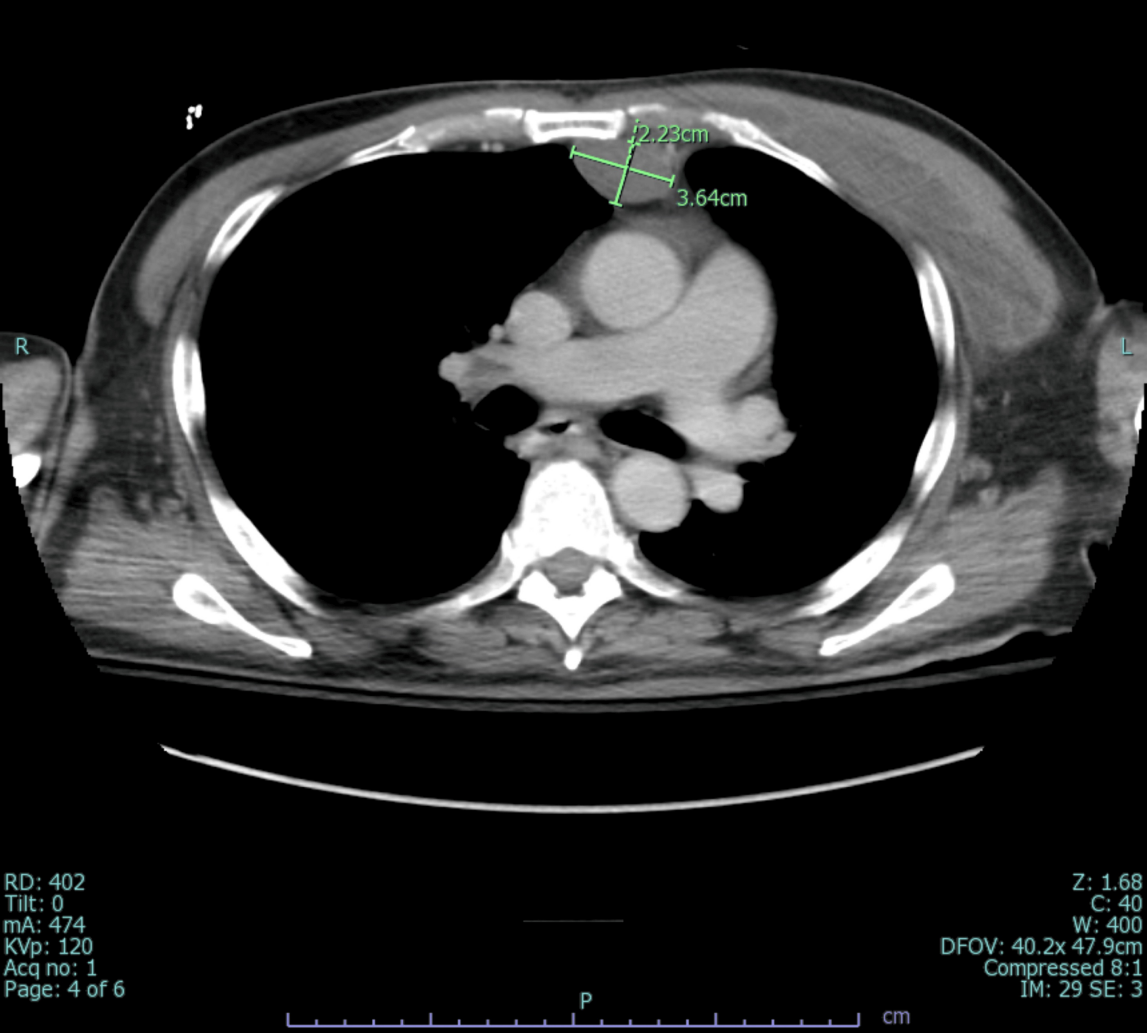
Computed tomography scan (axial view) showing a lower anterior mediastinal abscess.
